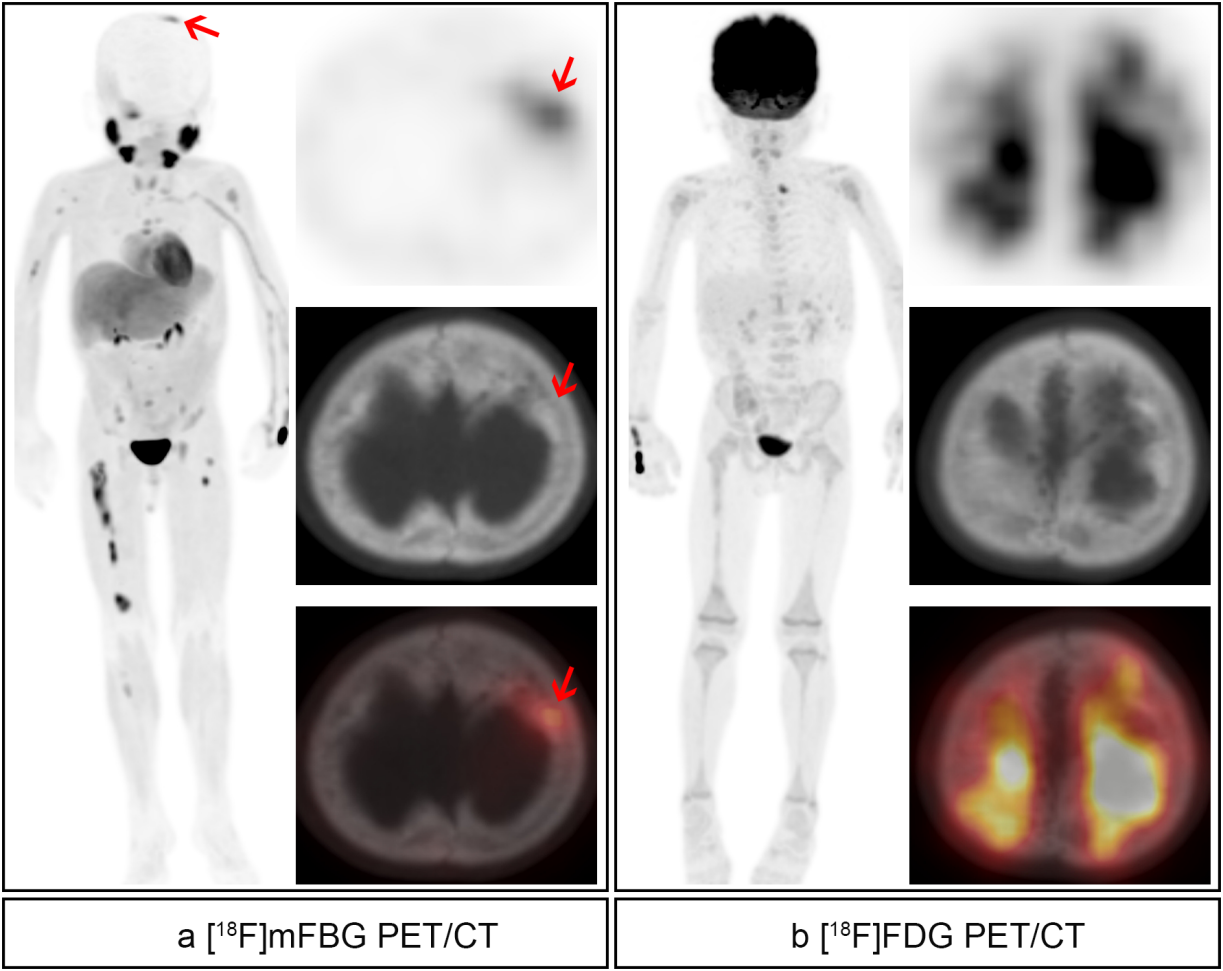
Therapy response evaluation of a 4-year-old boy with high-risk right retroperitoneal neuroblastoma underwent resection of lesions followed by 8 cycles of chemotherapy. (a) [^18^F]mFBG images. Pathological uptake was found in the skull (red arrows). (b) [^18^F]FDG images. No residual activity was found in the same site.
